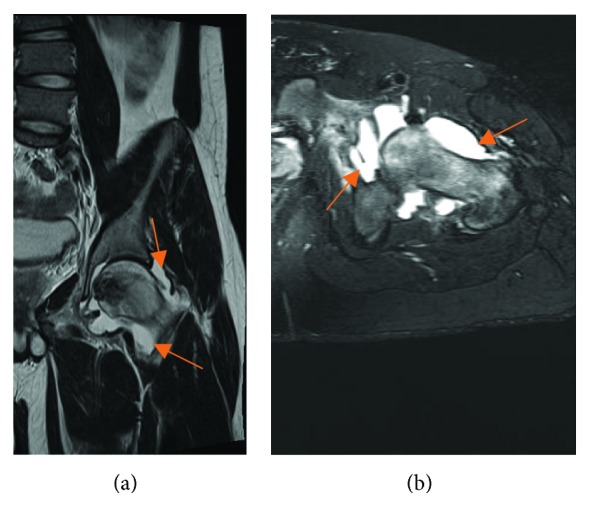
T2 MRI views of the left hip: coronal view (a) and axial view (b) of the hip with joint effusion (orange arrows).
